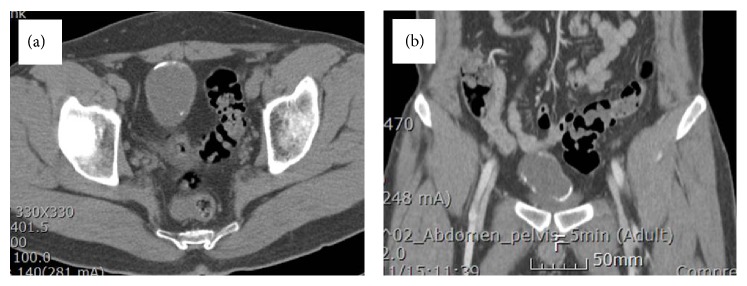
CT scan shows a lobulated cystic mass over the bladder dome which has calcified thick wall and is located at midline to the right side.
